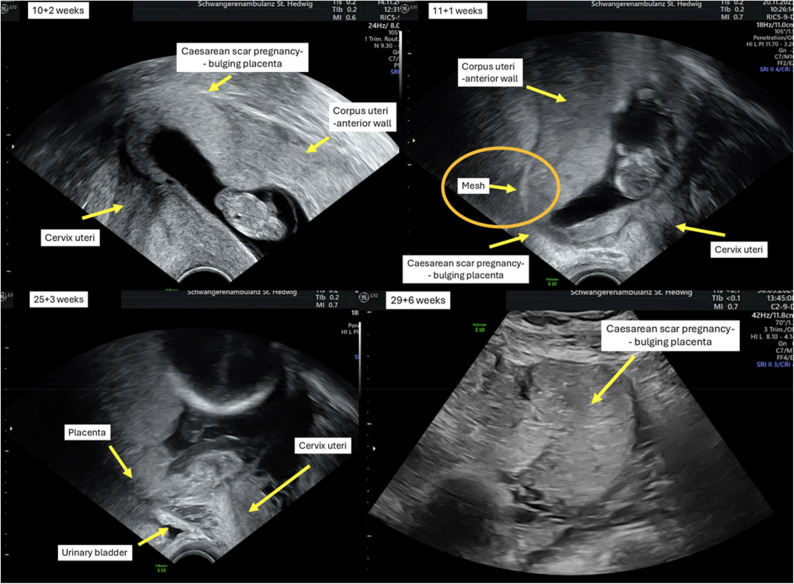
Transvaginal ultrasound images demonstrating uterine wall defects and implanted mesh
